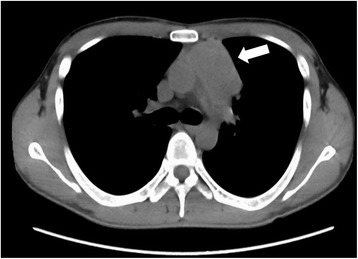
Computed tomography scan confirms a solid tumor (53×35 mm) in the anterior mediastinum (*arrow*)
